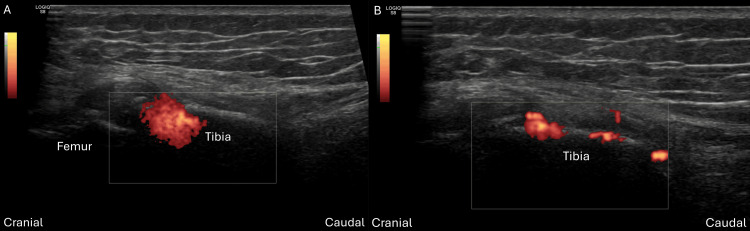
(A) The ultrasound (US) image in power Doppler imaging (PDI) mode showed a compact, ball-shaped Doppler hotspot. (B) After needle repositioning, the subsequent US image in PDI mode demonstrated a thin, sheet-like Doppler blush spreading evenly along the undersurface of the cortical line.
